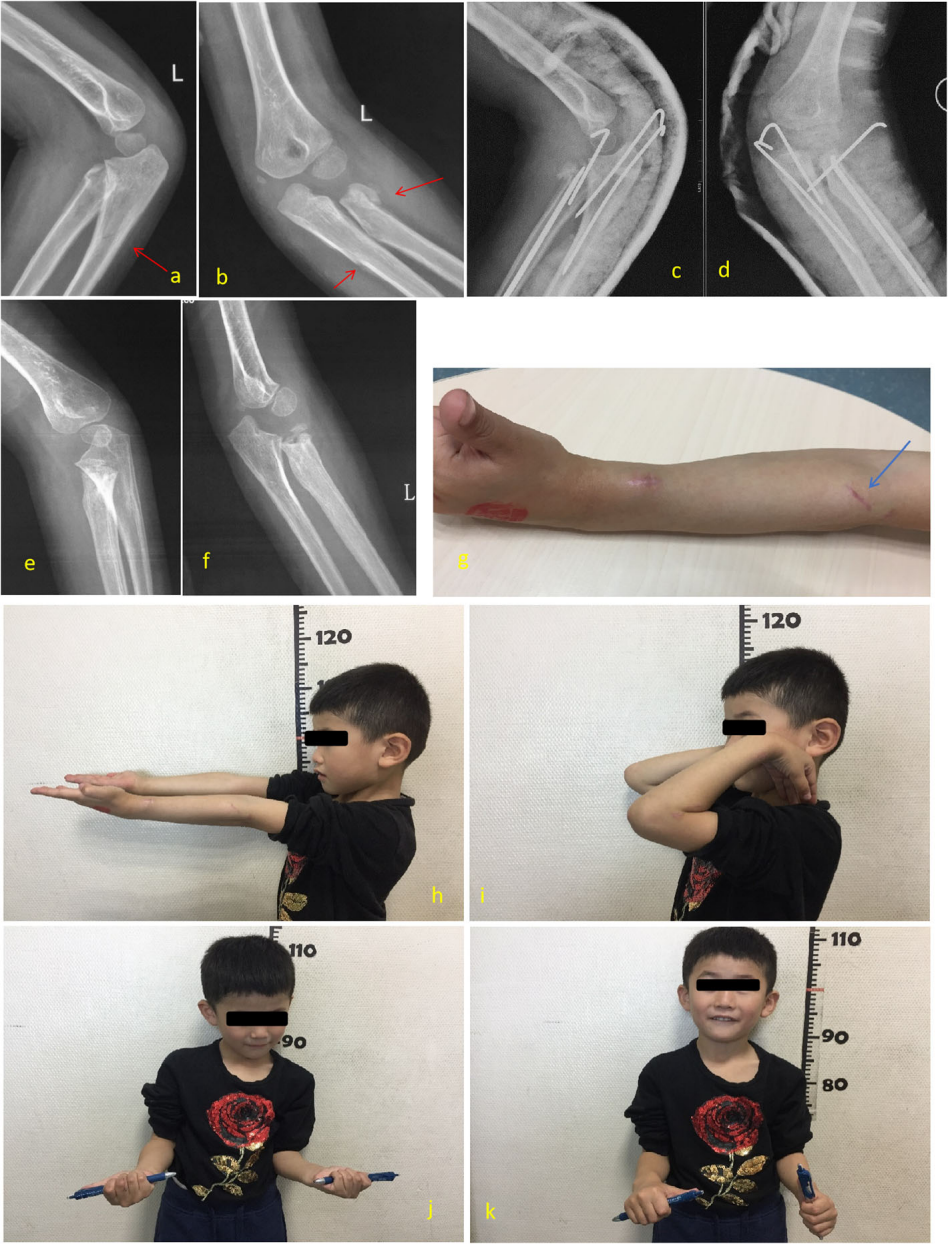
A 5-year-old boy classified type II injury in right elbow. a, b Lateral and A-P view of X-ray film show oblique through ulna coronoid process without displacement of ulna (arrow) associated with radial neck fracture with angulation more than 60°. c, d Lateral and A-P view of X-ray film show this boy was treated with close reduction and internal fixation with K-wire for olecranon, but open reduction and internal fixation with K-wires and ESIN for radial neck. e, f Six months postoperation, A-P and lateral view of X-ray film show the olecranon and radial neck fracture healed with ischemic change of radial head after K-wires and ESIN removed 3 months postoperation. g Mini incision for open reduction of radial neck fracture (arrow). h–k Twelve months postoperation, the flexion, extension, and supination were normal but pronation was limitated in left forearm
